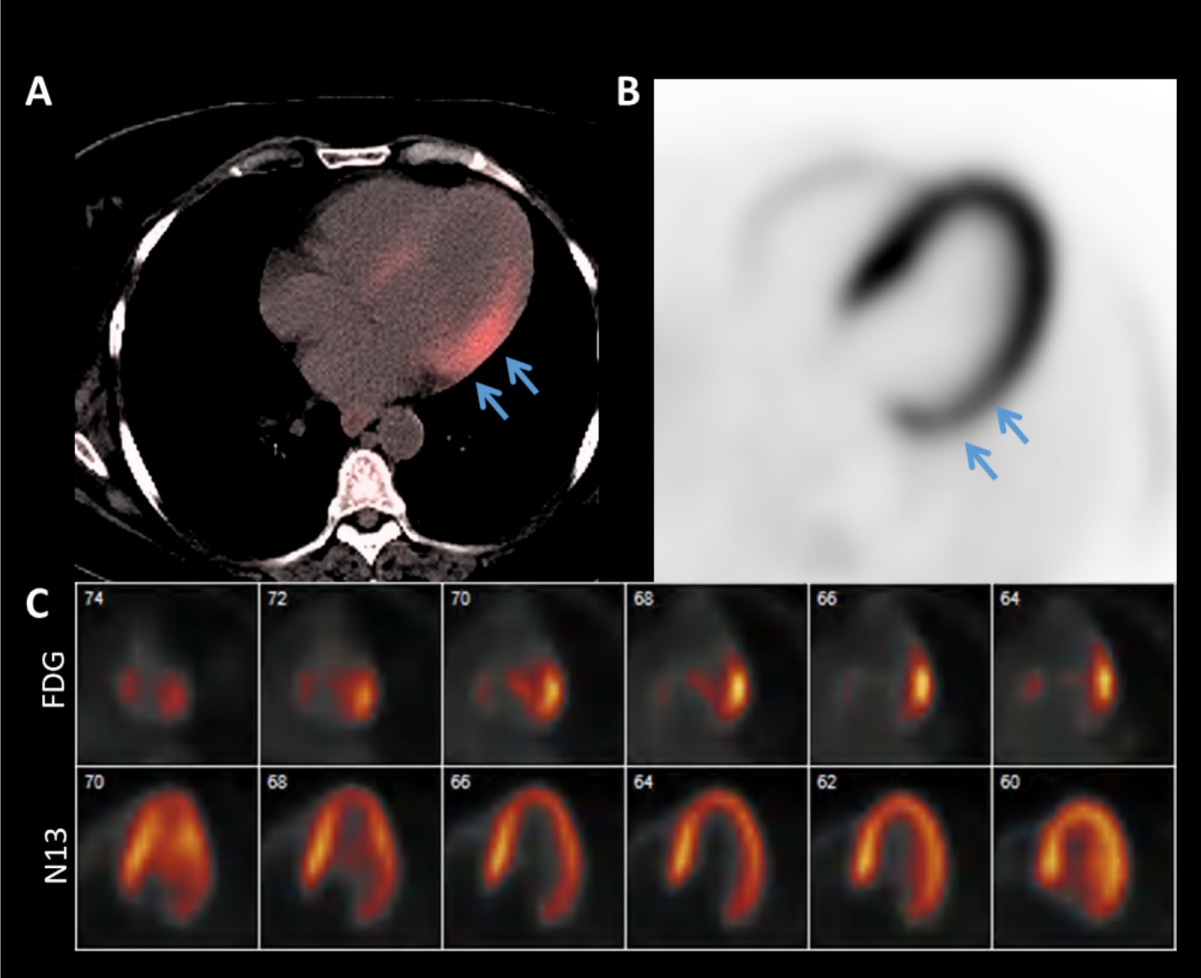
^18^F-fluorodeoxyglucose Positron Emission Tomography in Active Cardiac Sarcoidosis. An example of mismatched pattern in active sarcoidosis with ^18^F-fluorodeoxyglucose (FDG) uptake (A) and ^13^N-ammonia (N13)perfusion defect (B) in the basal to mid lateral wall (*blue arrows*). A horizontal long axis side by side comparison (C) of FDG uptake and N13 perfusion. From left to right, the views go from posterior to anterior
